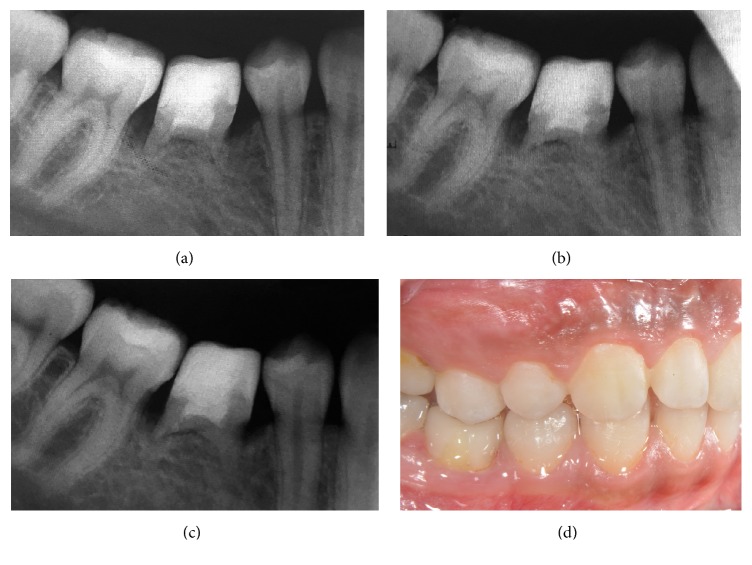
Follow-up views: (a) 1-year follow-up radiograph; (b) 2-year follow-up radiograph; (c) 3-year follow-up radiograph; (d) 3-year follow-up intraoral view.
